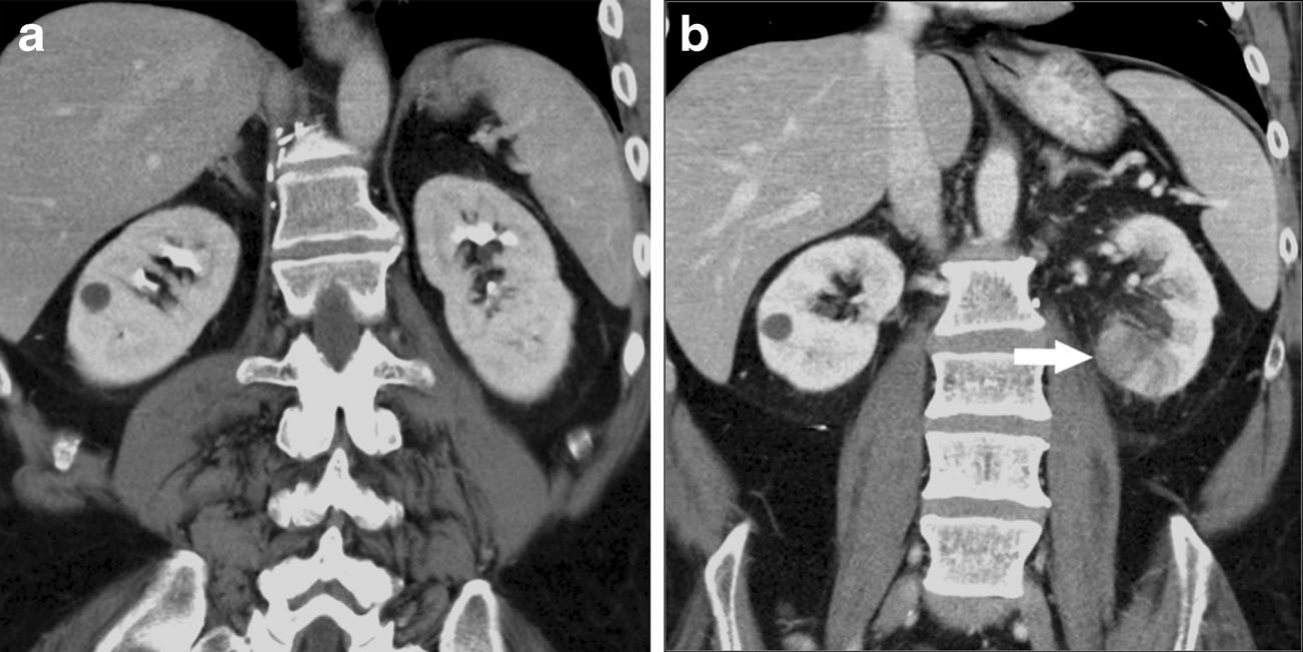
Fifty-four-year-old male with testicular cancer presenting with evidence of renal infarct following four cycles of bleomycin, etoposide, and cisplatin. (a) Pre-treatment coronal CT showing normal appearance of the kidneys. (b) Post-treatment coronal CT showing developing left renal infarct appearing as an area of hypoattenuation in the inferior aspect (*arrow*)
